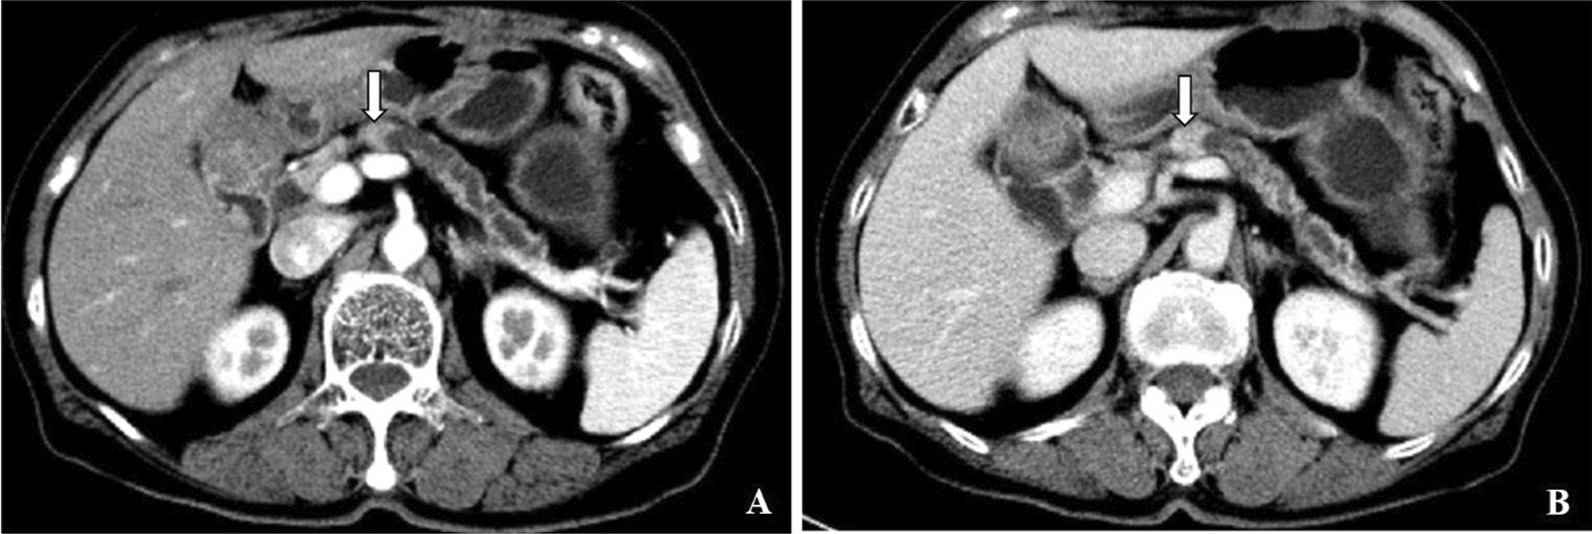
A 73-year-old woman with no discomfort and was admitted to our hospital for pancreatic mass detected by CT examination. Contrast-enhanced CT images show a mass in pancreatic head (arrow) with diameter 1.4-cm, hyperdense in A arterial phase and B portal venous phase. Tumor infiltration and enlarged lymph nodes are absent. NLR is 1.12. Patient was alive for 40 months until our last follow-up after standard pancreaticoduodenectomy with no tumor recurrence
